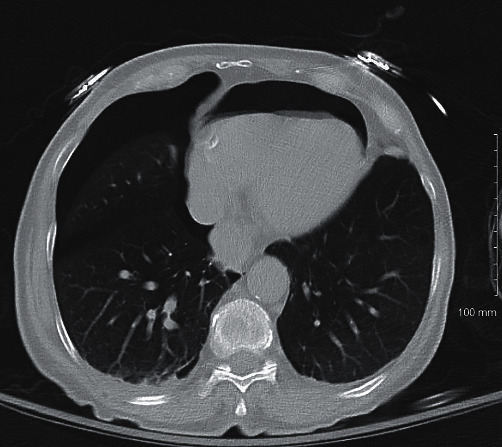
Noncontrast chest CT scan showing pneumopericardium, pneumothorax, and pericardial effusion.
